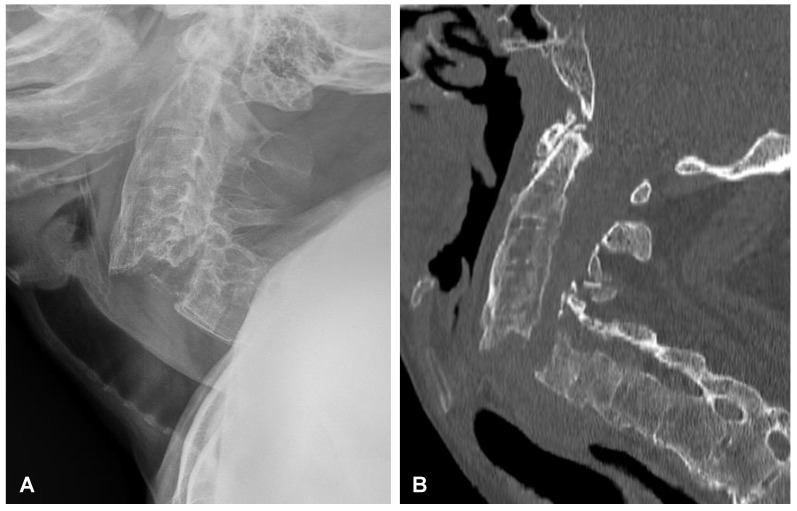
Preoperative plain radiograph (A) and CT scan (B) of the cervical spine demonstrating unstable extension transdiscal three-column fracture at the C5/C6 level.
